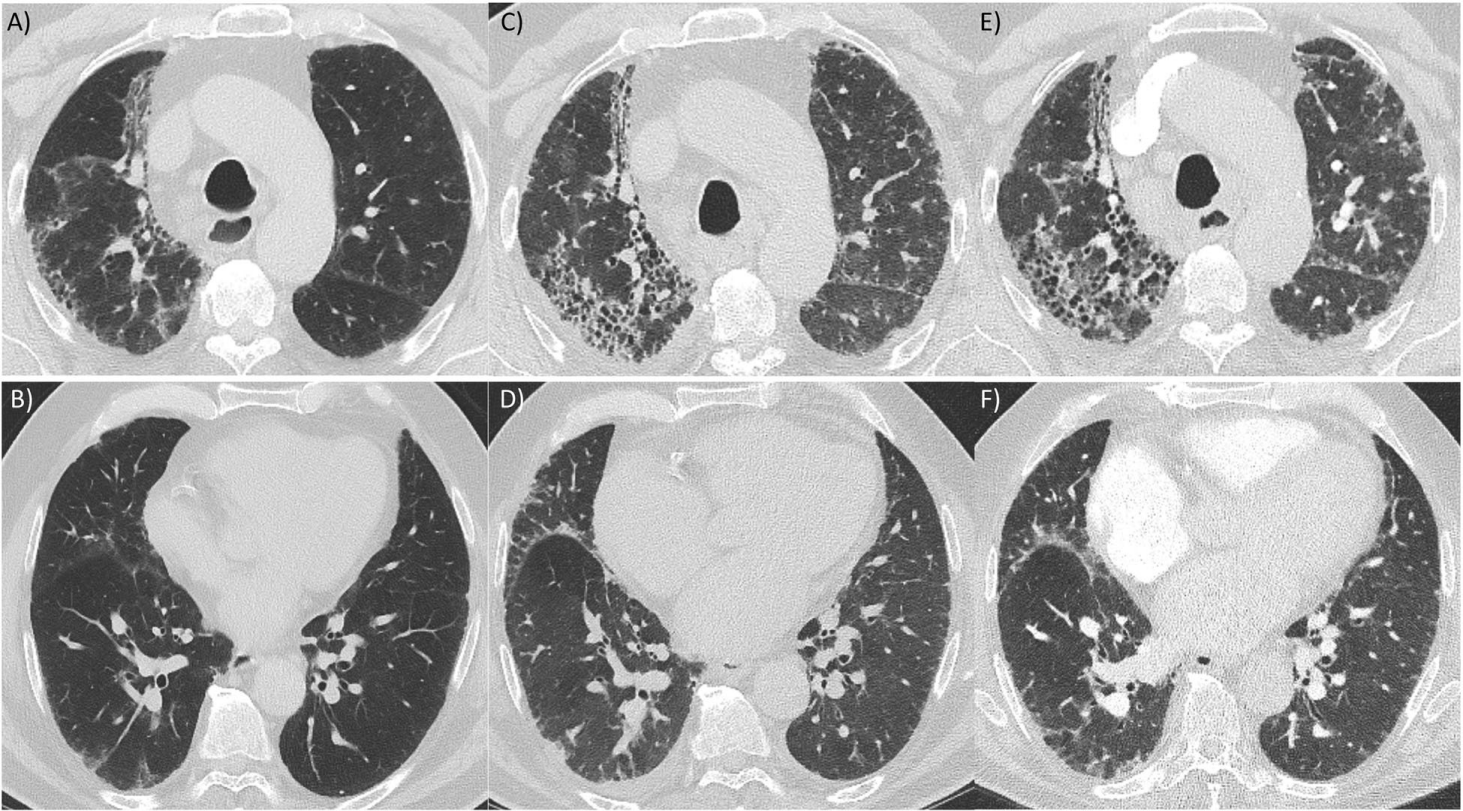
Computed tomography imaging of the chest in a patient with progressive unclassifiable interstitial lung disease. Serial apical (a, c, e) and basal (b, d, f) axial images at baseline (a, b); at 36 months (c, d); and at 42 months (e, f). Images show upper lobe predominant pulmonary fibrosis with progressive reticulation, traction bronchiectasis, and honeycombing
